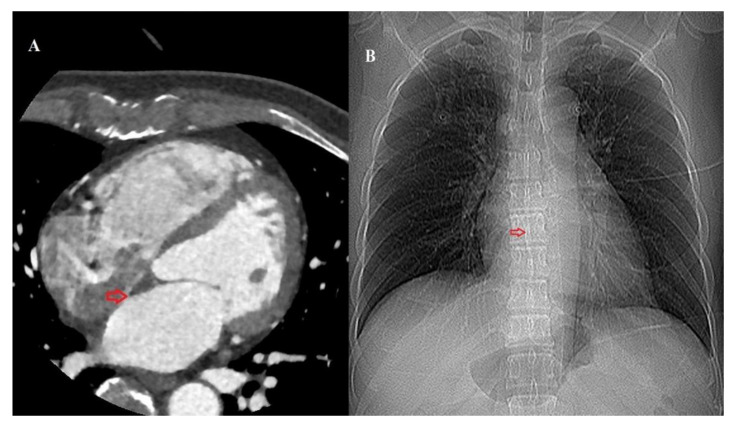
(A) Axial multidetector-row computed tomography (CT) image of the heart identifying the fossa ovalis (red arrow). (B) The location of the fossa ovalis is marked on the corresponding CT scout image.
